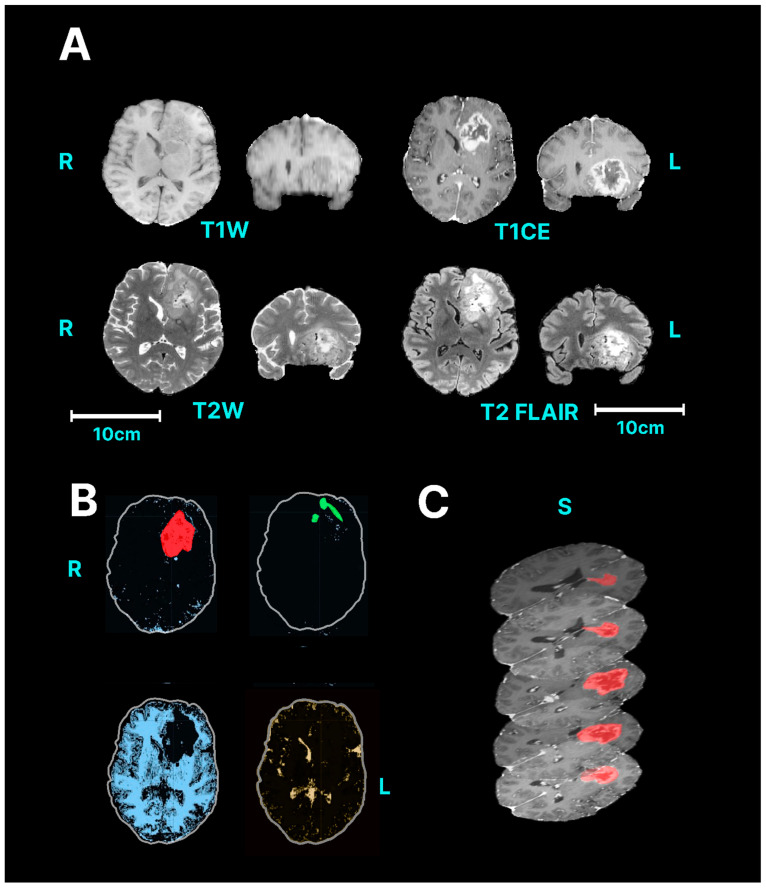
Semi-automated segmentation of a glioblastoma. (A) Original MRI. (B) Capturing the extent of GBM through classification. Red represents glioblastoma, green represents cerebral oedema, blue represents brain parenchyma and yellow represents cerebrospinal fluid. (C) Derivation of 3D lesion volume. (Single column with colour in print).
